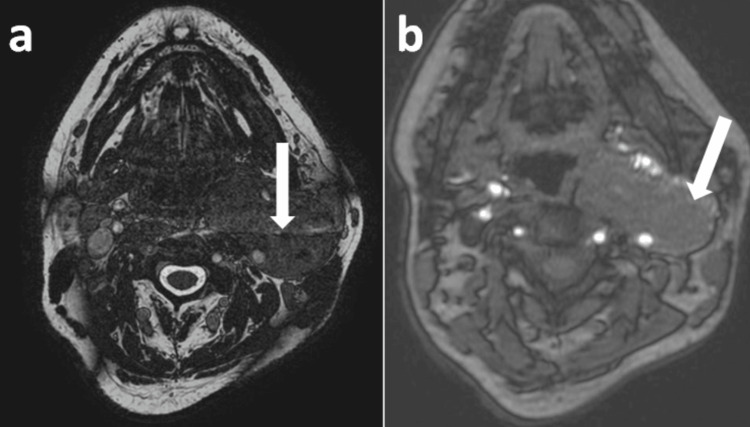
MRI images of the neck demonstrating a lesion in the left carotid space. (a) MRI T2-weighted image axial section showing hyperintense lesion (long white arrow) epicentered in the left carotid space. (b) Magnetic resonance angiography (MRA) neck image axial section showing muscle isointense lesion (long white arrow) epicentered in the left carotid space causing splaying of ECA and ICA. ECA: external carotid artery; ICA: internal carotid artery
